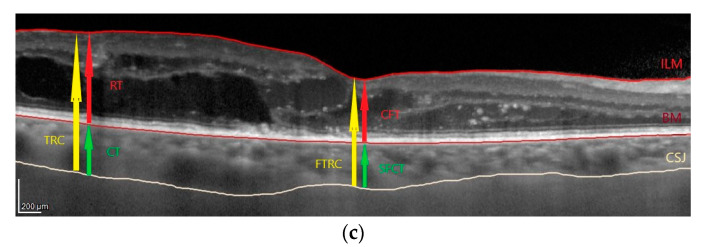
Representative OCT scans across the fovea representing measurements and types of diabetic macular oedema (DME): (a) control; (b) cystoid; (c) diffuse. DME with subretinal fluid was classified as such regardless of concomitant cystoid or diffuse oedema. In cases of mixed cystoid and diffuse oedema, the eye was classified according to the predominant type. As described previously [37], internal limiting membrane (ILM) and Bruch’s membrane (BM) were detected automatically, while the choroidal-scleral junction (CSJ) was marked manually. Subfoveal choroidal thickness (SFCT) was defined as the distance between the BM and the CSJ at the fovea (green arrow). The SFCT was manually measured using the calliper tool and calculated as the difference between the foveal total thickness (FTRC, retinal + choroidal thickness, from the ILM to the CSJ; yellow arrow) and the central foveal thickness (CFT; red arrow). Analogically, the choroidal thickness outside the fovea (CT; green arrow) was calculated by subtracting the retinal thickness (RT; red arrow) from the total thickness (TRC, retinal + choroidal thickness, from the ILM to the CSJ, yellow arrow).
